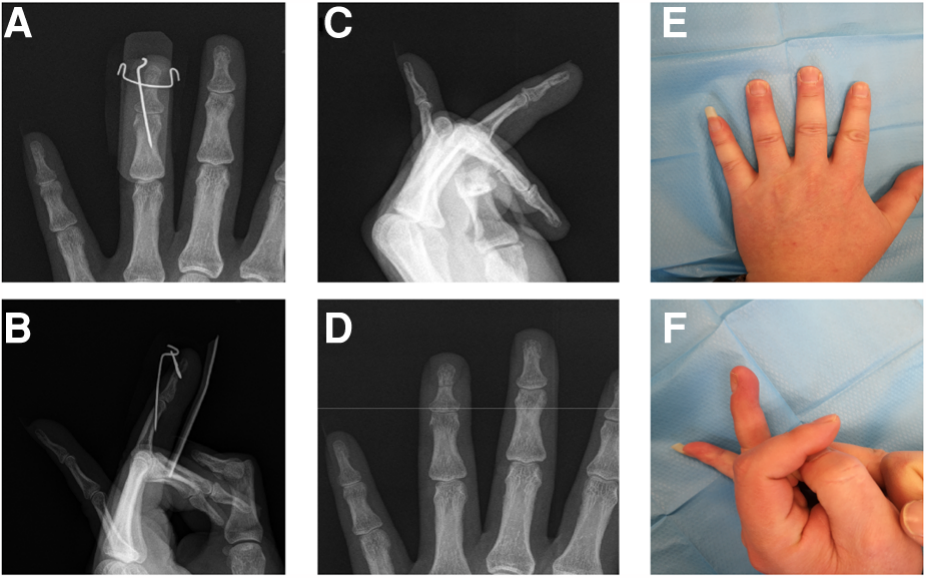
Follow-up of patient No. 4. (A,B). X-ray at 6 weeks postoperatively. (C,D). X-ray at 22 weeks postoperatively. (E,F). Finger appearance at 22 weeks.
